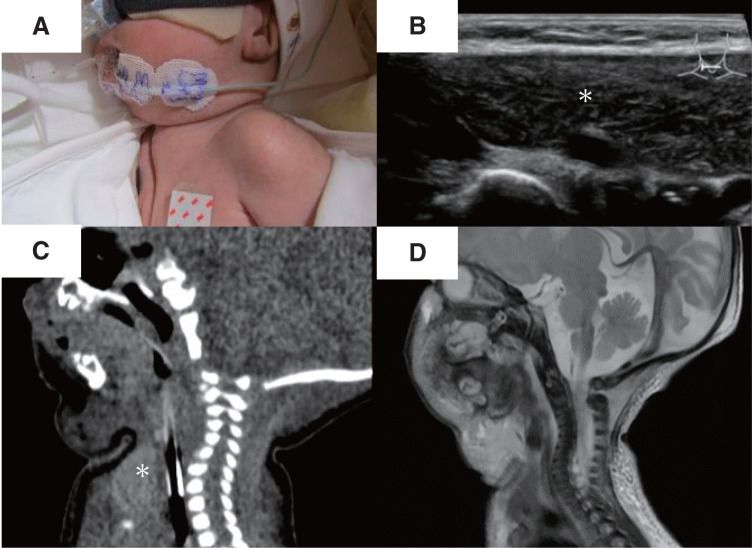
(A) A mass intermittently visible at the midline of the neck during exhalation, particularly when crying. (B) Axial ultrasonographic image of the suprasternal region, showing the thymus (∗) protruding superiorly into the neck. (C) Sagittal CT image and (D) T2-weighted MR image, both showing slight cephalad protrusion of the thymus (∗). CT, computed tomography; MR, magnetic resonance
